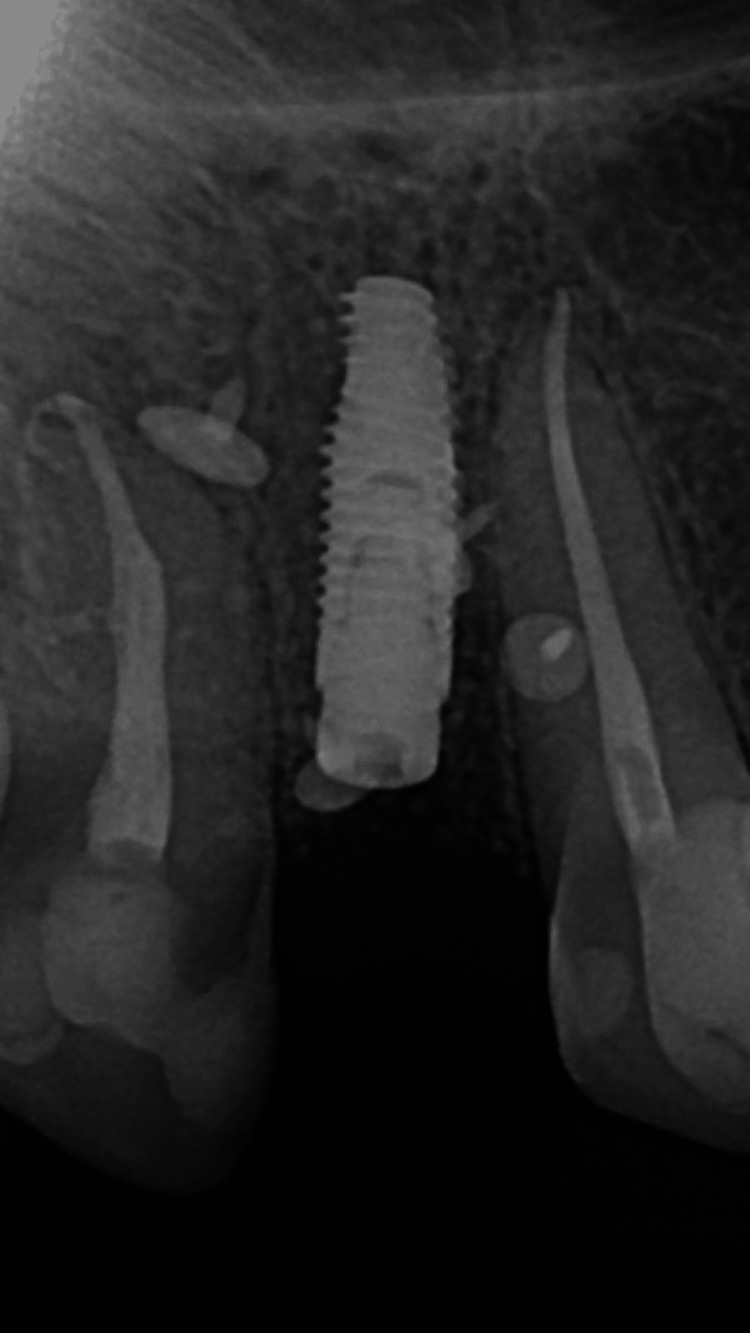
Periapical X-ray following implant placement and guided bone regeneration Titanium pins used to secure the collagen membrane are visible around the implant.
